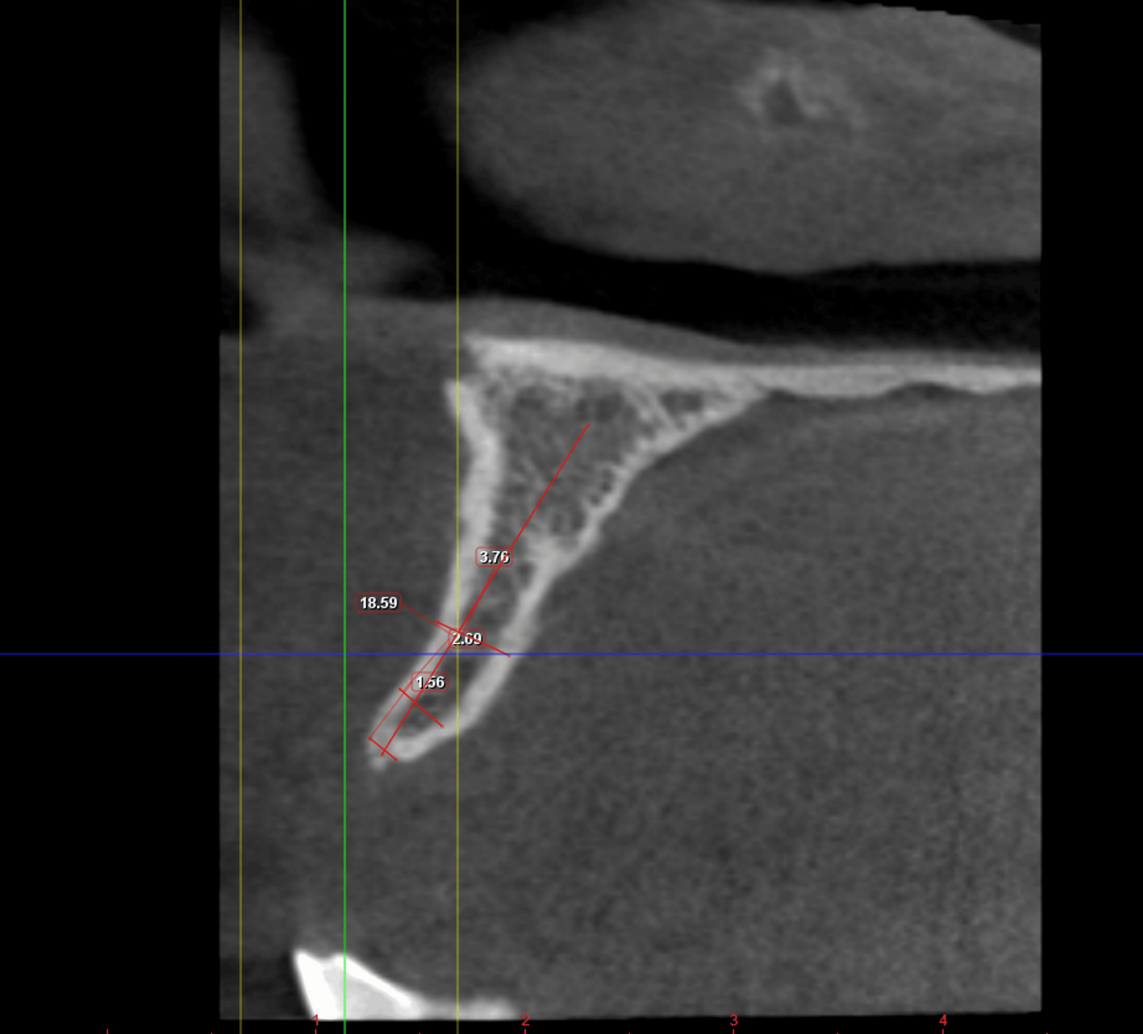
Case 3: The coronal section of the CBCT image of the patient showing 1.56 mm B-L width at the crest, 2.69 mm in the middle third, and 3.76 mm at the apical region of 21 region, which is inadequate for implant placement using conventional osteotomy B-L: Buccolingual.
